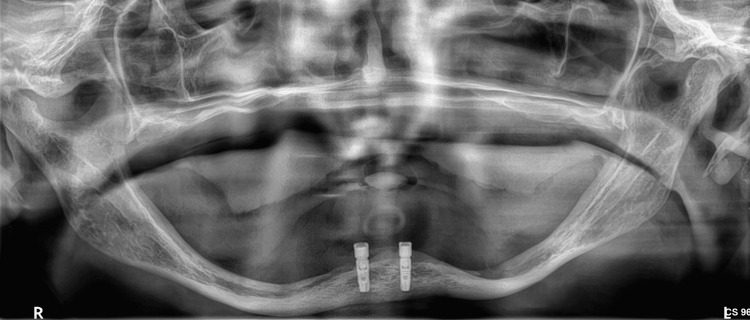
Panoramic X-ray of a 71-year-old female patient. Notice the extreme resorption on the maxilla and the mandible (Class VI in the Cawood and Howell classification)
